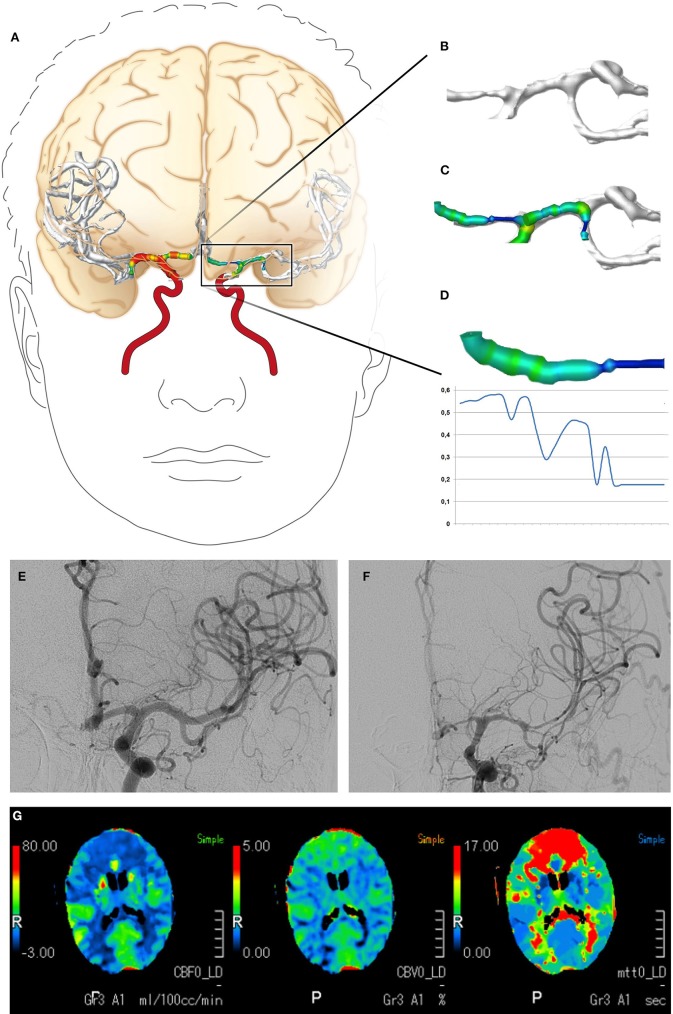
Digital reconstruction and volumetric analysis of the cerebrovascular tree from CTA data, the classification of vasospasm from DSA data, and the evaluation of CTP. (A–D) The volumetric evaluation of CTA data. The cerebrovascular tree was virtually reconstructed and a volumetric analysis of the defined vessel segments was performed. (E,F) Examples of vasospasm scoring. The vessel diameters of the A1 and M1 segments declined by >50%, resulting in vasospasm scores of 2. (G) Example of CTP with cerebral hypoperfusion (elongated mean transit time, reduced cerebral blood flow) in both anterior cerebral artery territories. CTA, computed tomography angiography; DSA, digital subtraction angiography; CTP, computed tomography perfusion imaging.
